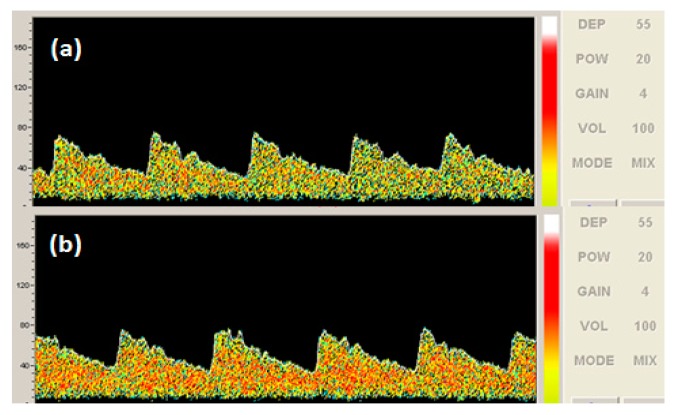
The Doppler sonographic measurement at 20% power: (a) commercial transducer; (b) PMN-PT single crystal based transducer.
